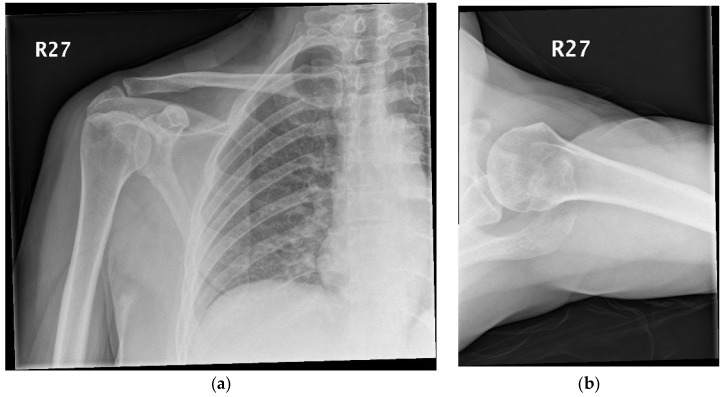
(a,b) Radiographs of the right shoulder.
